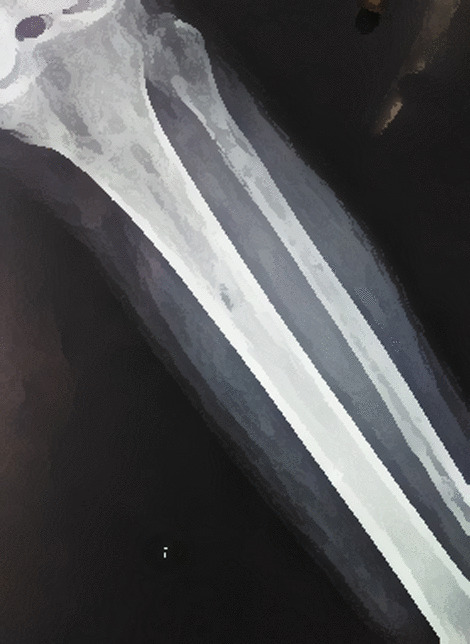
Anteroposterior x-ray of the patient’s left tibia and the fibula. Initial x-rays upon arrival, indicating an osteolytic lesion of the diaphysis
